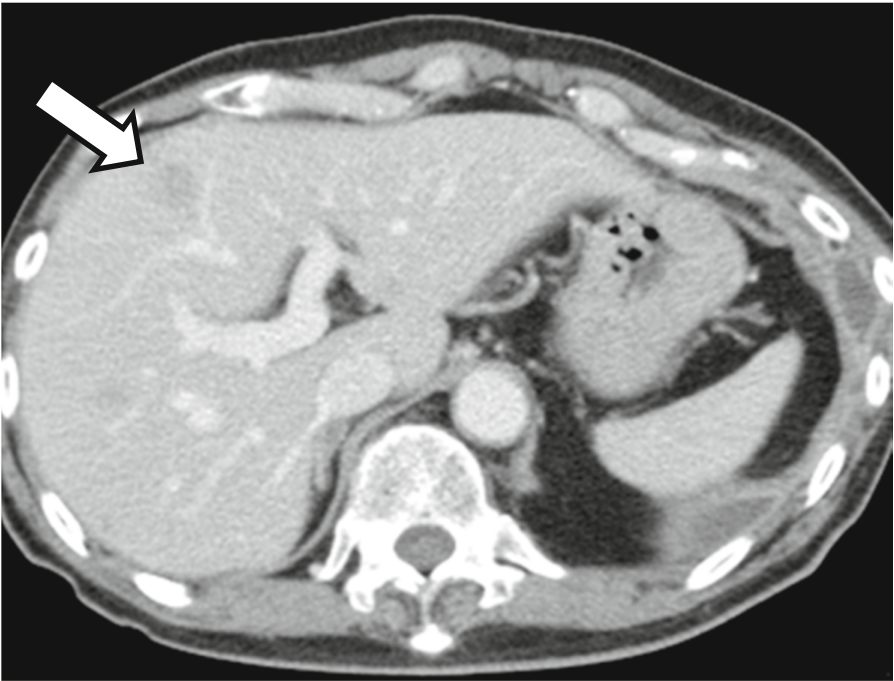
Enhanced computed tomography (CT) imaging. CT was performed after a single course of cytotoxic chemotherapy. The size of the liver metastasis in the S4 region increased from 1.0 to 1.3 cm (arrow)
